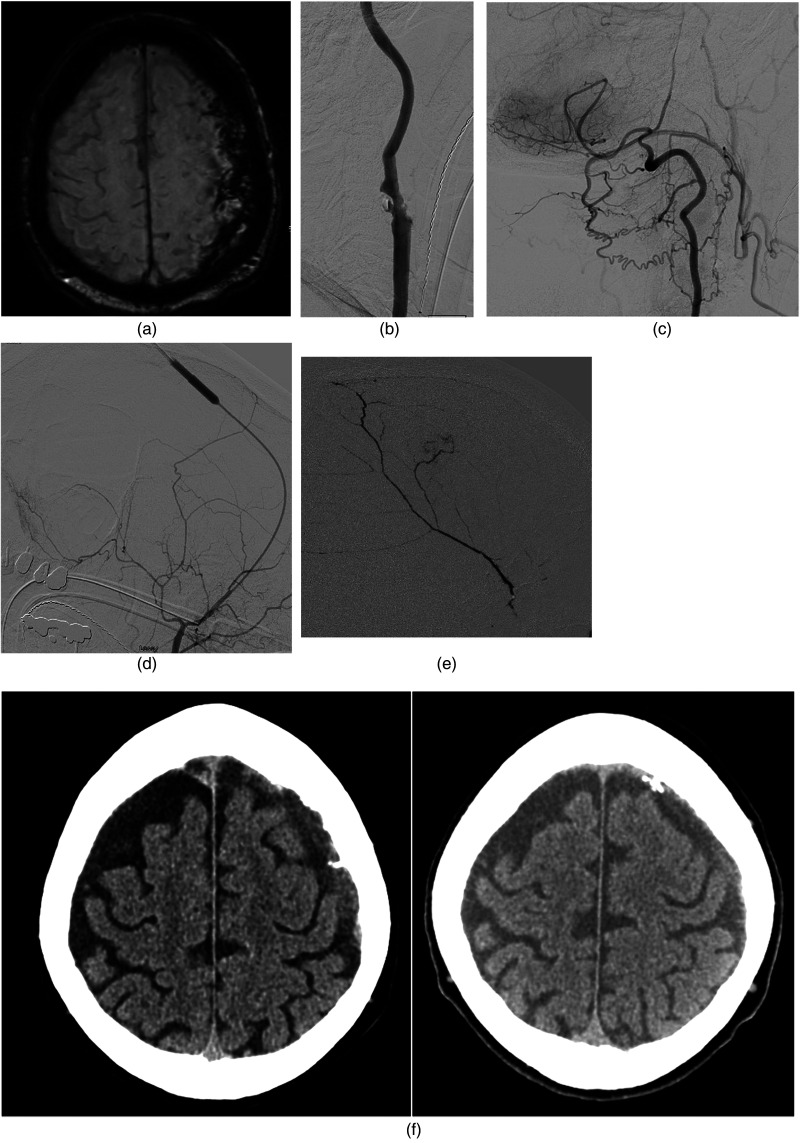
(a) Bilateral cSDH with a thick 2 cm left-sided cSDH was presumably the cause of neurological deficits via mass effect. (b) Complete occlusion of the left ECA precluded routine access to the MMA. (c) Angiography of the left vertebral artery revealed muscular collaterals feeding the left occipital artery which flowed in a retrograde fashion to fill the ECA territory, verifying patency of the STA and MMA. (d) Headway Duo 167 cm (Microvention) was advanced over an 0.014” microwire (Stryker) to reach the target location. (e) Successful right-sided MMA embolization with 9:1 nBCA (Cerenovus) via the Headway Duo 167 cm microcatheter (Microvention) was performed resulting in satisfactory penetration of the territory. (f) Follow-up CT 2 weeks (left image) and 1 month postprocedure (right image) revealed reduction of the cSDH to 8 and 5 mm in thickness. cSDH: chronic subdural hemorrhage; CT: computed tomography; ECA: external carotid artery; MMA: middle meningeal artery; STA: superficial temporal artery.
